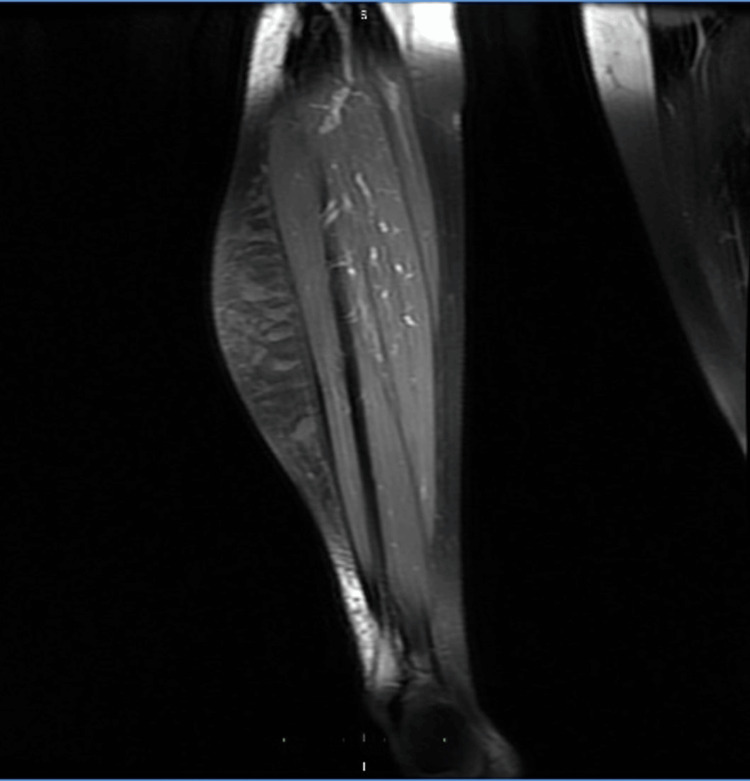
Coronal T1 view showing the lesion in the intermuscular plane which appears as a low signal on T1-weighted imaging and shows homogenous suppression. The fat plane is maintained with the adjacent muscles.
